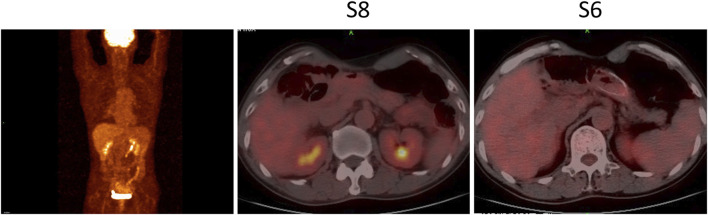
PET-CT in August 2020 showed disappeared hypermetabolic activities in the hepatic lesions and no clear sign of tumor recurrence and metastasis throughout the body. S6, Segment 6; S8, Segment 8.
